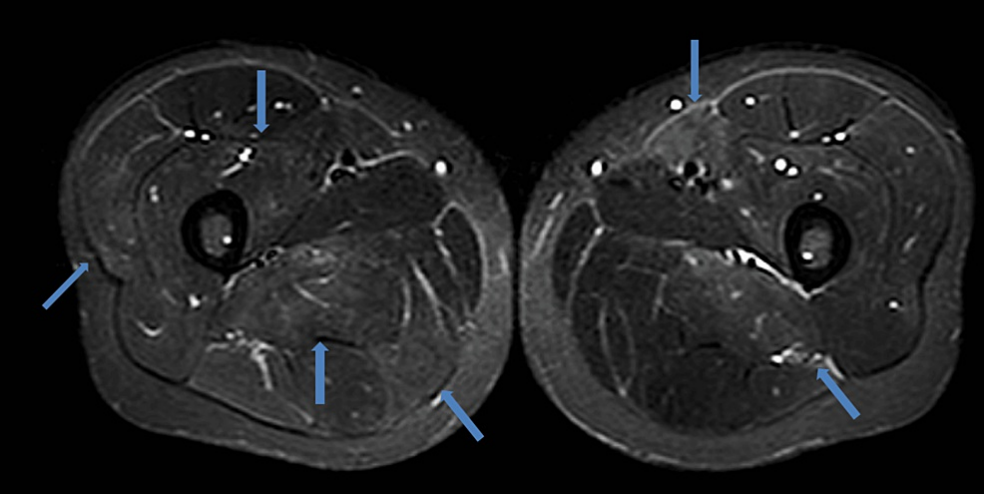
Magnetic resonance imaging short TI inversion recovery of the thighs (transverse view) Magnetic resonance imaging short TI inversion recovery of the thighs (transverse view) showing a weak, heterogeneous high signal in the fascia and the muscles of the abductor, adductor, quadriceps, and hamstring muscles of both thighs (blue arrows).
